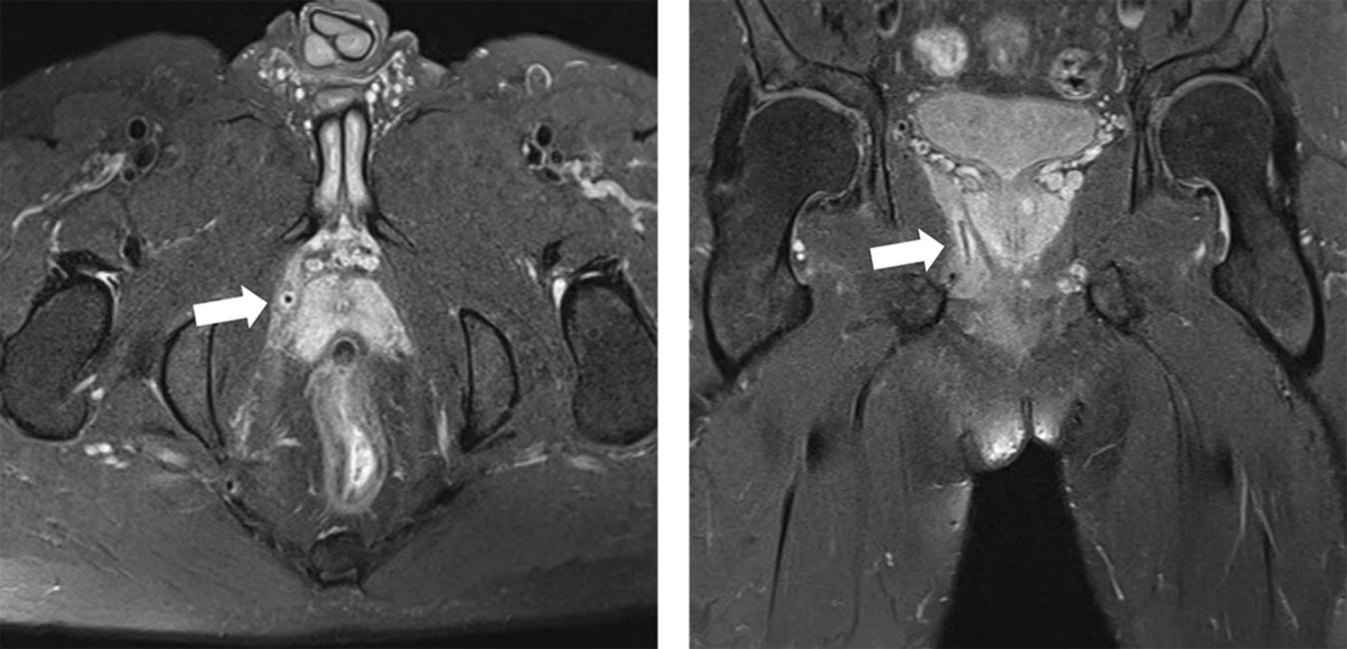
Preoperative perianal magnetic resonance imaging shows the long fistula tract (white arrow) located near the prostate and under the levator ani muscle
